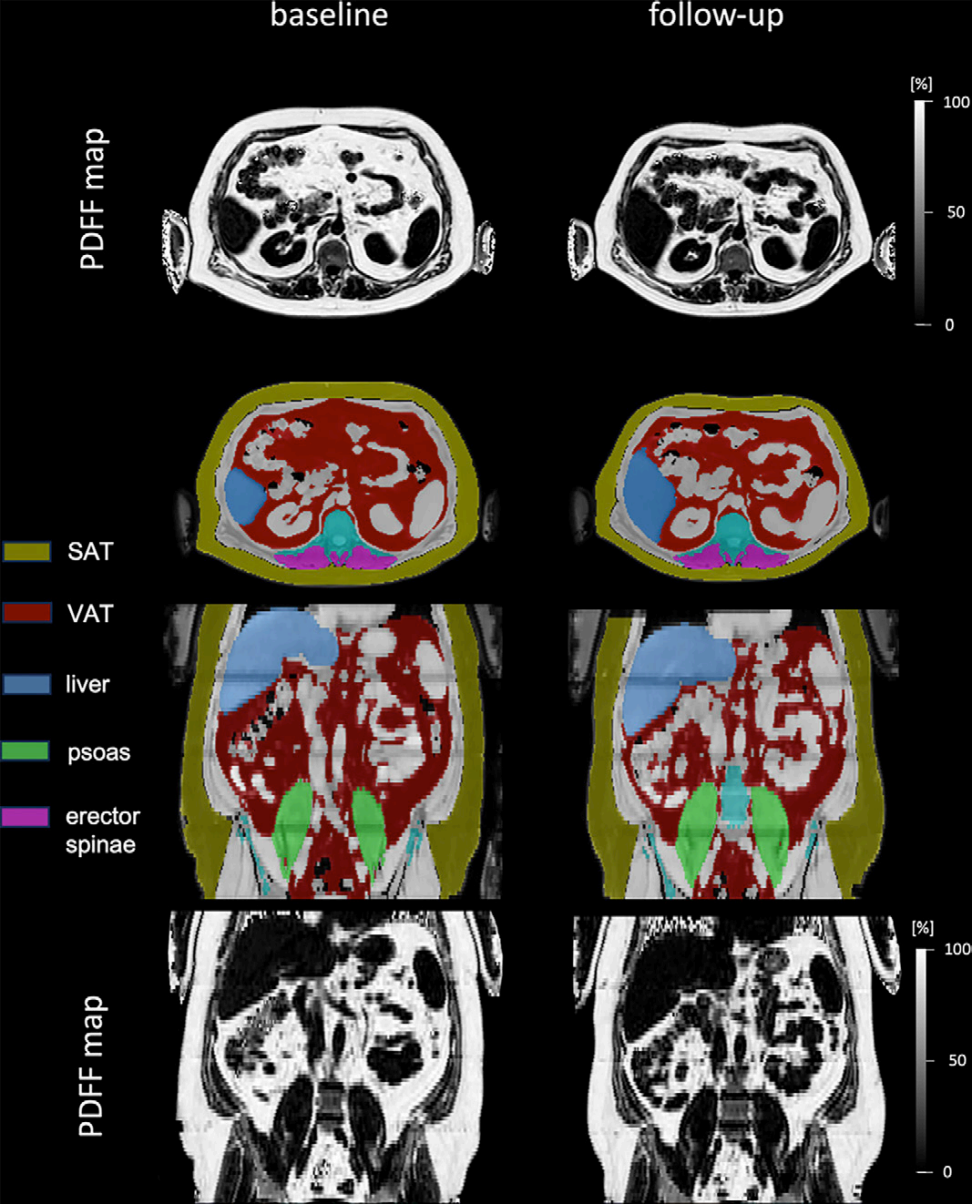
Axial and coronal view of MRI-based proton density fat fraction (PDFF) maps
